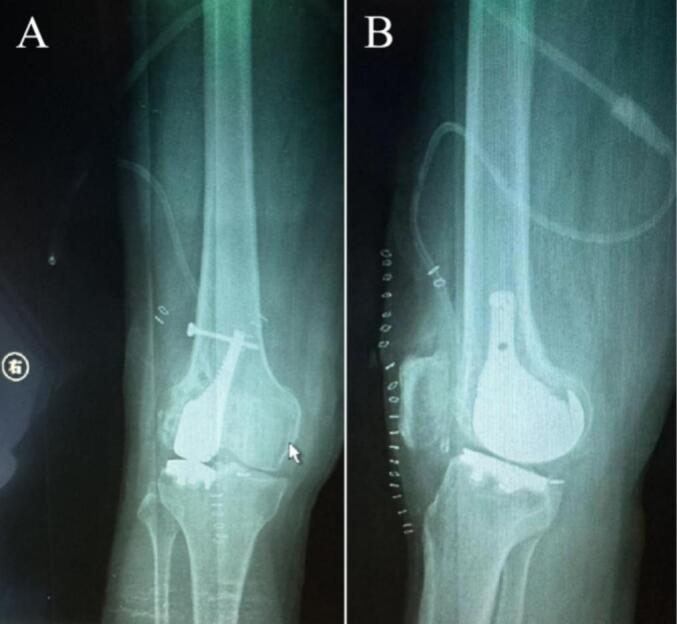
The intraoperative x-ray images. (A) AP view of the right knee. (B) Lateral view of the right knee.
